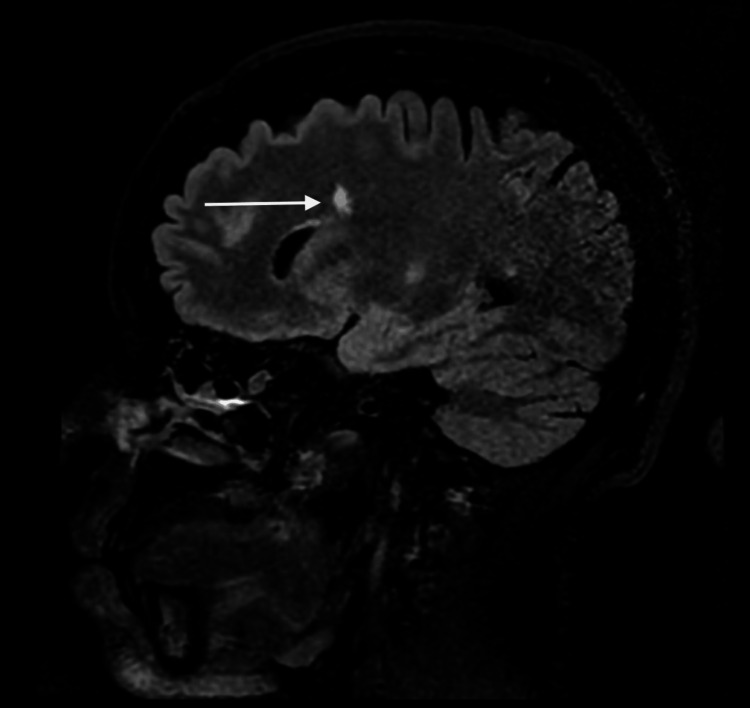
Brain MRI performed after the first seizure (sagittal section, FLAIR) Left paraventricular FLAIR intensity, with a long axis perpendicular to the ventricular axis (white arrow) MRI: magnetic resonance imaging; FLAIR: fluid-attenuated inversion recovery
